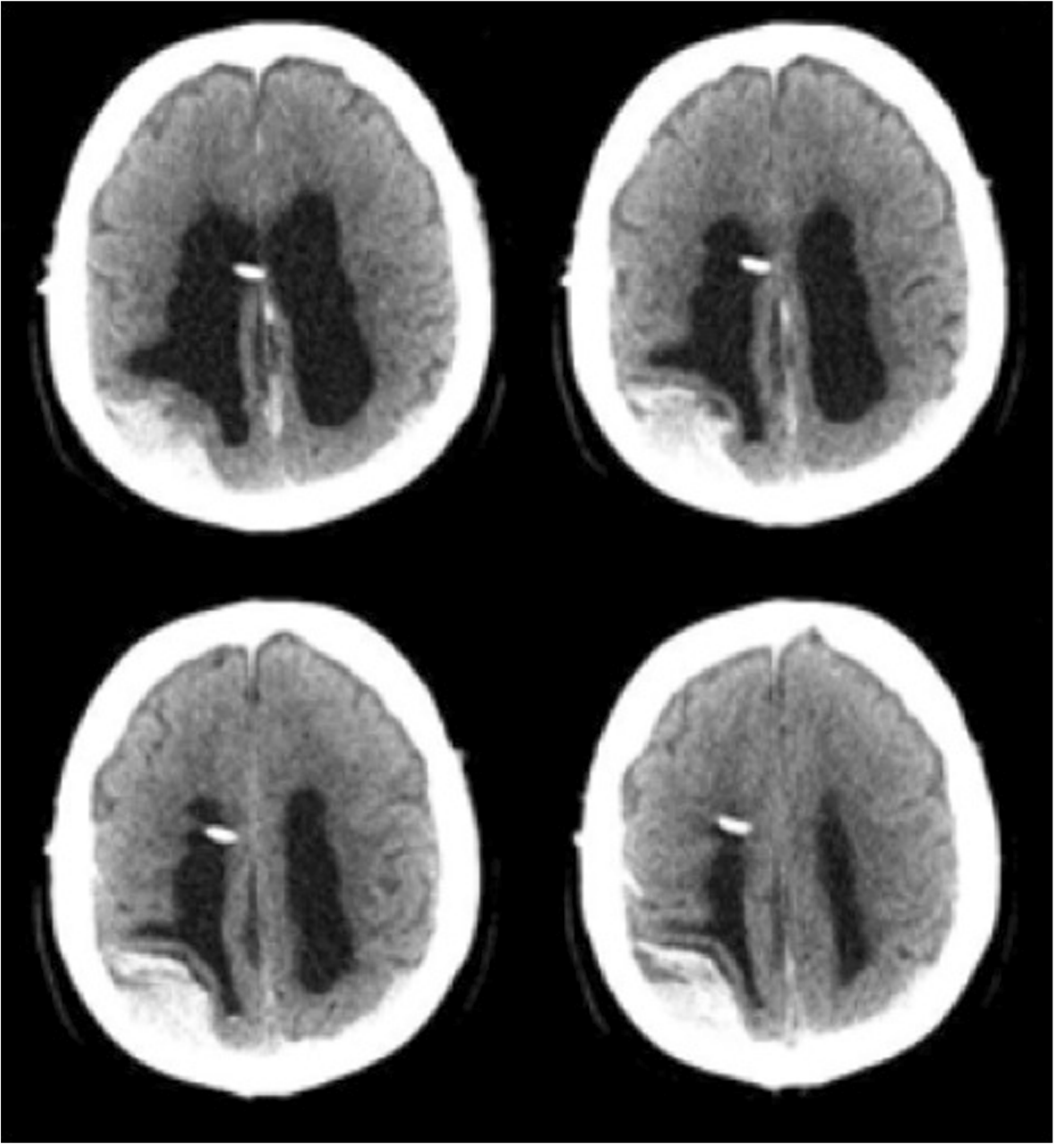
Pre-valve adjustment computed tomography scan. The pre-valve adjustment computed tomography scan showing a high-density subdural hematoma in the right convexity and a mixed density subdural hematoma in the left convexity.
